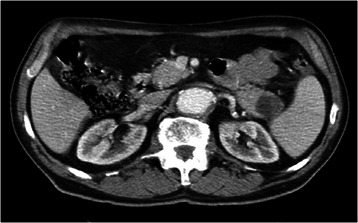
Abdominal CT with contrast: caudal pancreatic pseudocyst (after 2 months).
